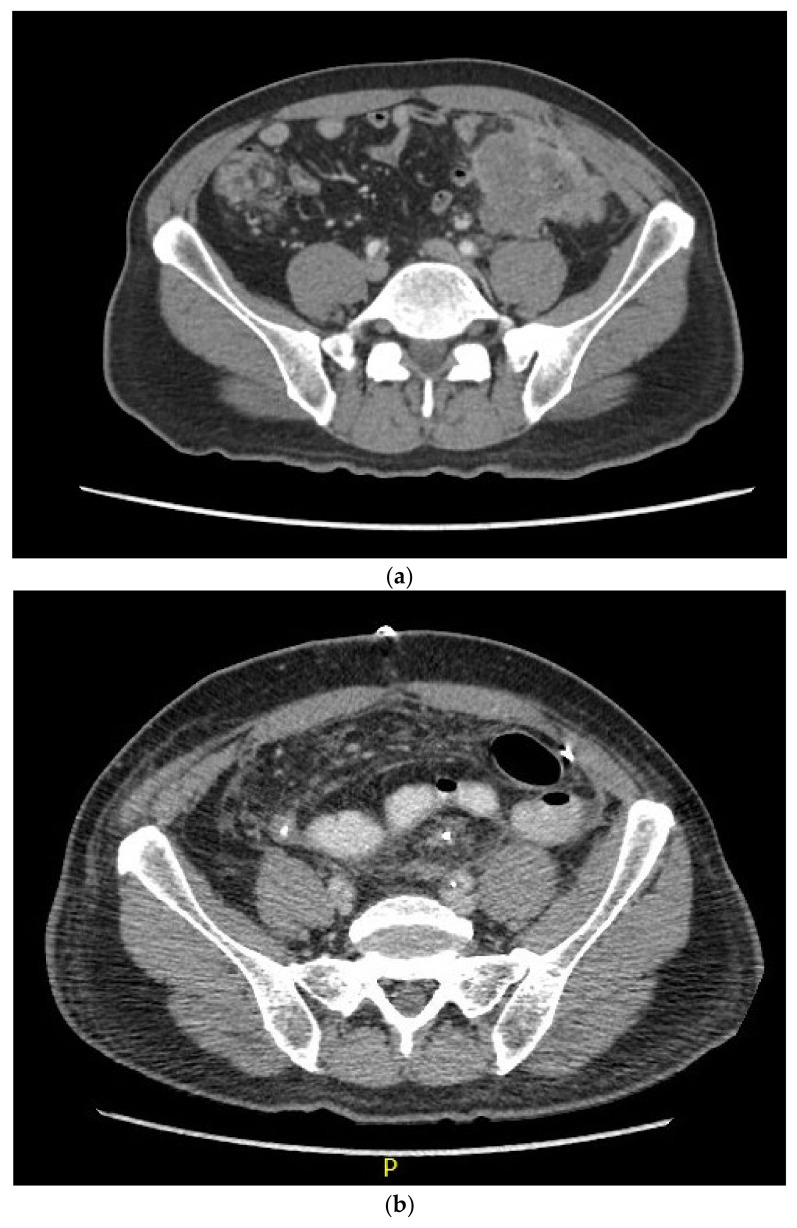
CT scans from (a) February 2021, pre-treatment, and (b) October 2021, pre-surgery.
